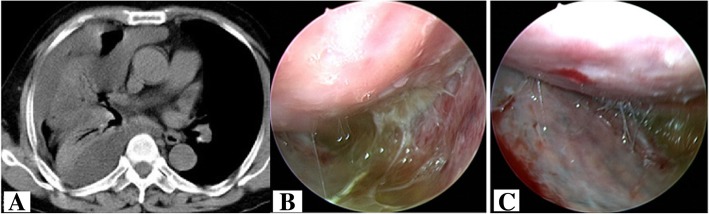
High-resolution computed tomography revealed pleural effusion (a). Thoracoscopy showed yellow pleural effusion and adhesions (b) and multiple small nodules (c) in the pleura
